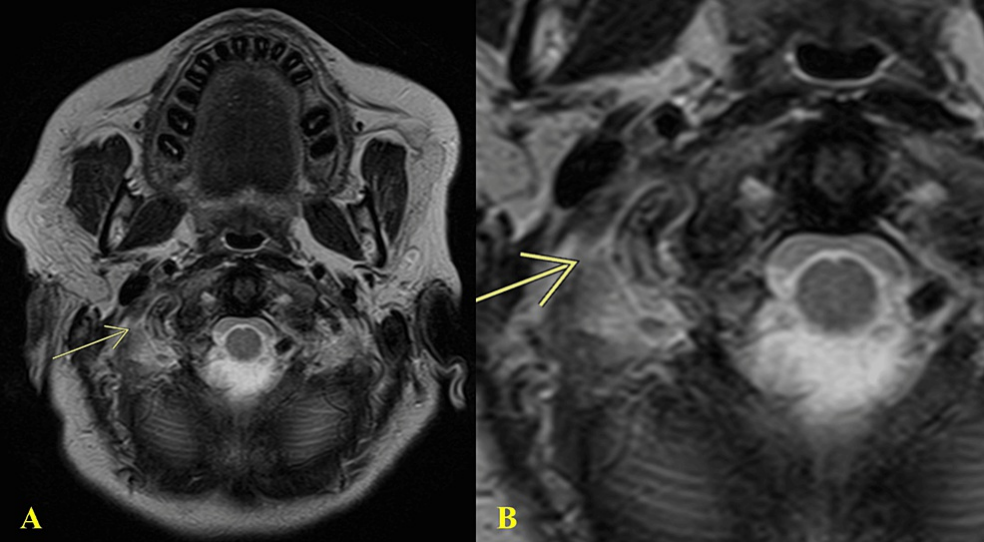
T2 axial MRI displays an impaired flow void of the distal right vertebral artery V3 segment representing known vertebral artery dissection and hematoma, measuring about 1.8 cm in length (A). A magnified image better depicts impaired flow void (B) MRI: magnetic resonance imaging
